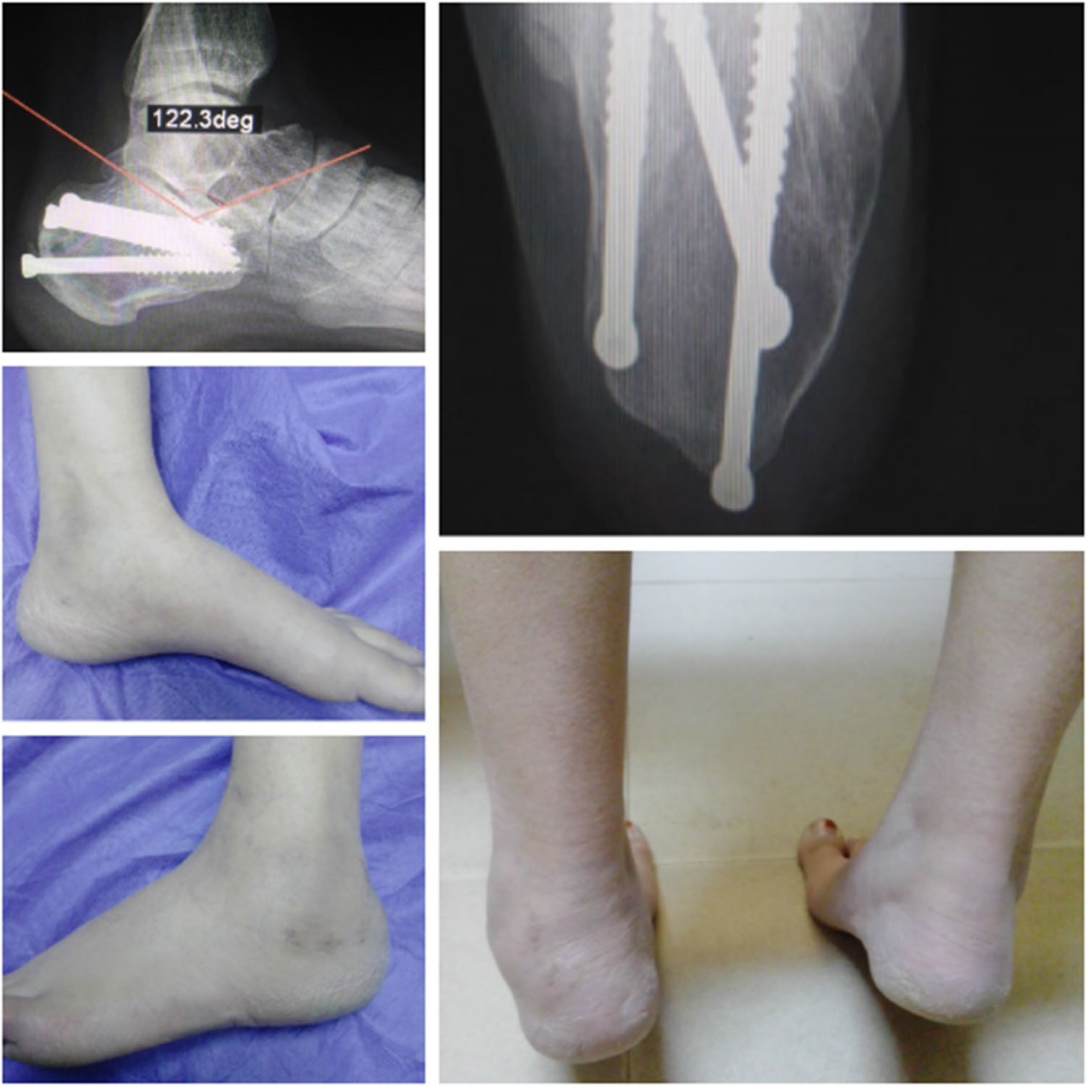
Final radiographic and clinical follow-up of patient with cannulated screw fixation
